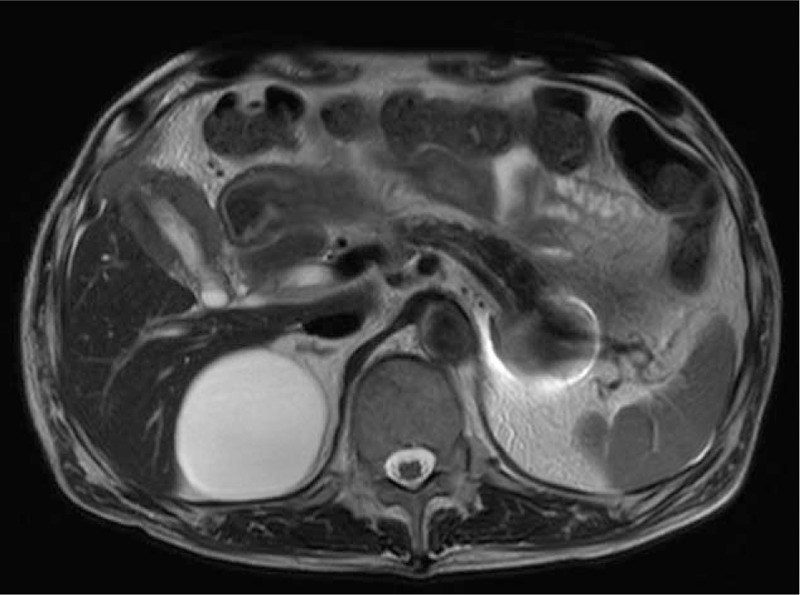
T2-weighted MR imaging revealed homogeneous thickening wall structure with hyposignal intensity. Gallbadder stones were not observed.
